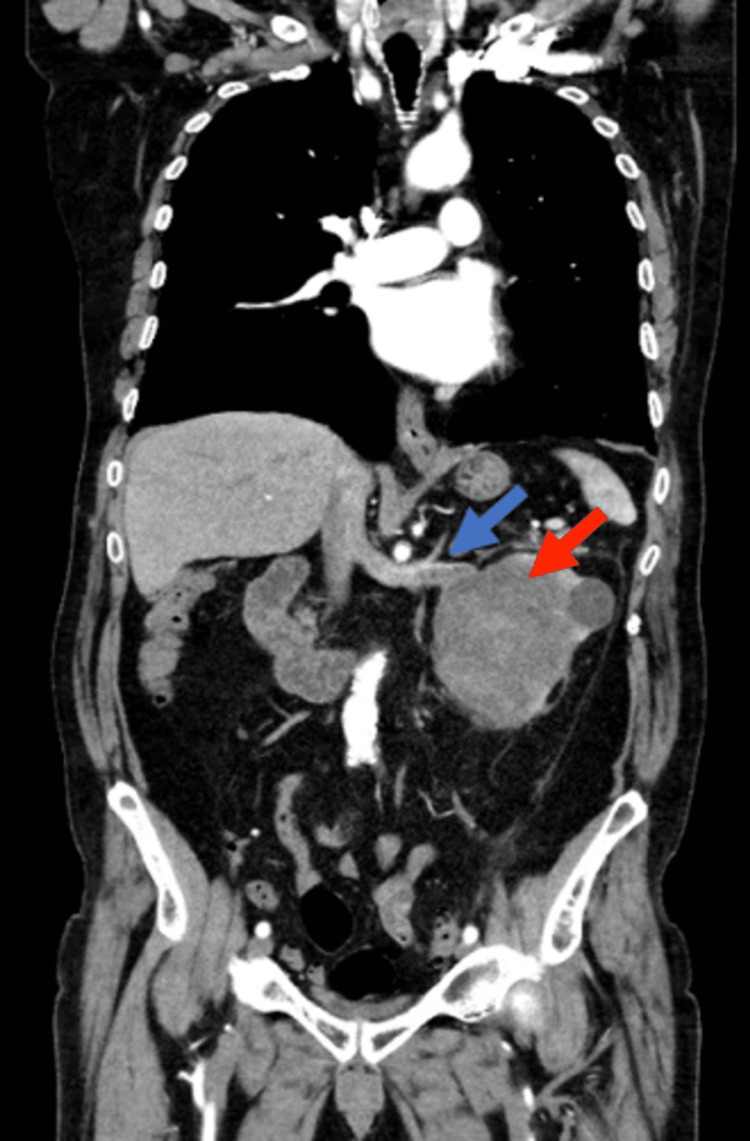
CT scan coronal view at presentation CT scan coronal view showing left renal vein tumor thrombus (blue arrow) and large renal tumor with 8cm diameter (red arrow). CT: Computed tomography
